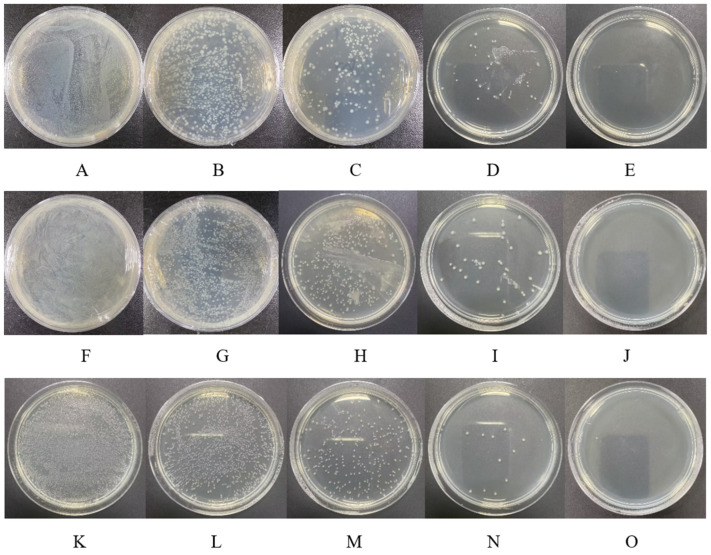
Plate coating results of bacterial solution at different times of ultrasonic crushing. (A–E): ds*Oftermicin1*-HT115 was crushed for 0 h, 0.5 h, 1 h, 1.5 h, and 2 h, respectively. (F–J): ds*Oftermicin2*-HT115 was crushed for 0 h, 0.5 h, 1 h, 1.5 h, and 2 h, respectively. (K–O): ds*GFP*-HT115 was crushed for 0 h, 0.5 h, 1 h, 1.5 h, and 2 h, respectively.
